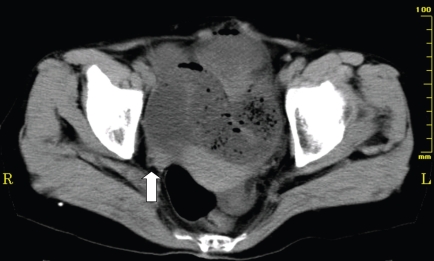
An abdominal computerized tomography (CT) showed largely distended small bowel loops and a smooth transition zone (arrow) at the site of obstruction.
